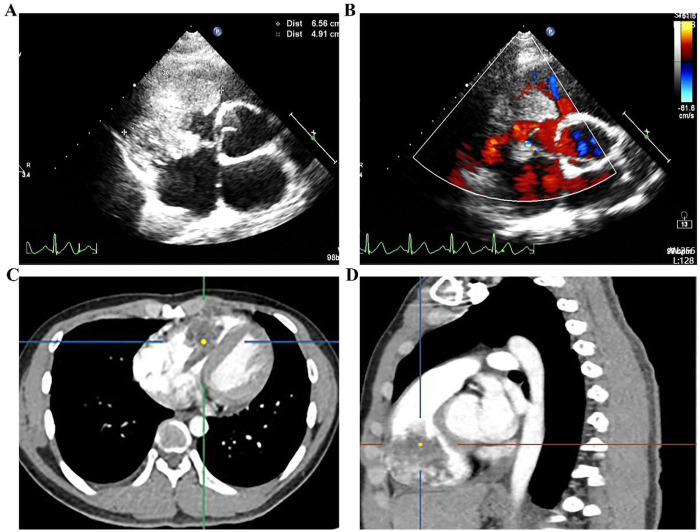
Echocardiography and enhanced chest CT demonstrating a right ventricular space-occupying lesion. (A,B) Echocardiogram; (C,D) enhanced chest CT.
